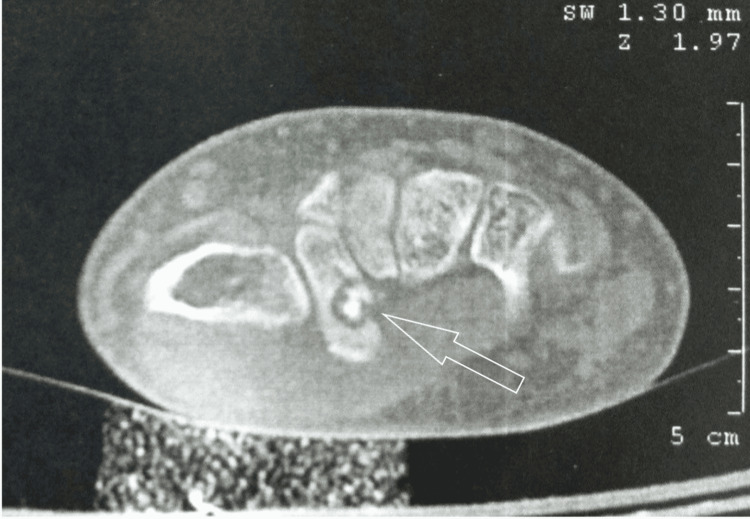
CT tomography Computed tomography reveals the characteristic dense nidus, surrounded by a radiolucent halo.
